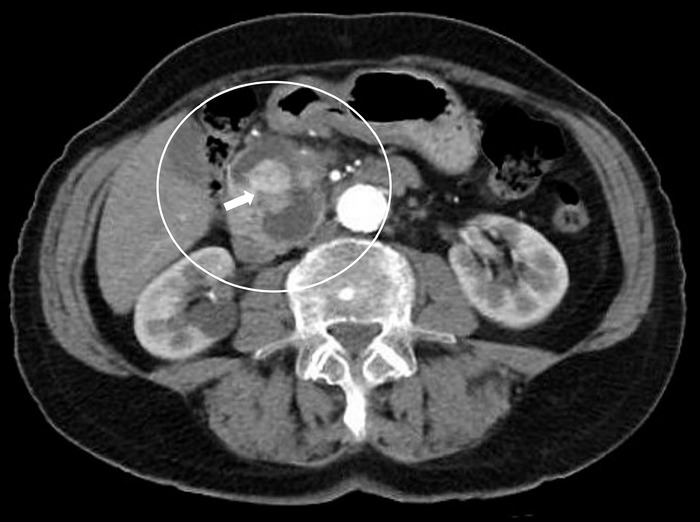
Contrast-enhanced axial abdominal computed tomography scan showed a 4.9 × 3.5 × 2.8-cm pancreatic cystic lesion in the uncinate process with a mural nodule in the cystic lumen (arrow).
